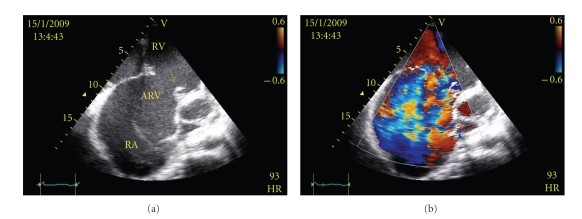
Transthoracic apical four-chamber view showing: (a) downward displacement of the septal leaflet of the tricuspid valve (arrow) in relation to the anterior mitral valve leaflet (MV), several enlargement of right atrium (RA), atrialized portion of right ventricle (ARV), and true right ventricle (RV); (b) severe tricuspid valve regurgitation due to the anomalous valvular arrangement.
